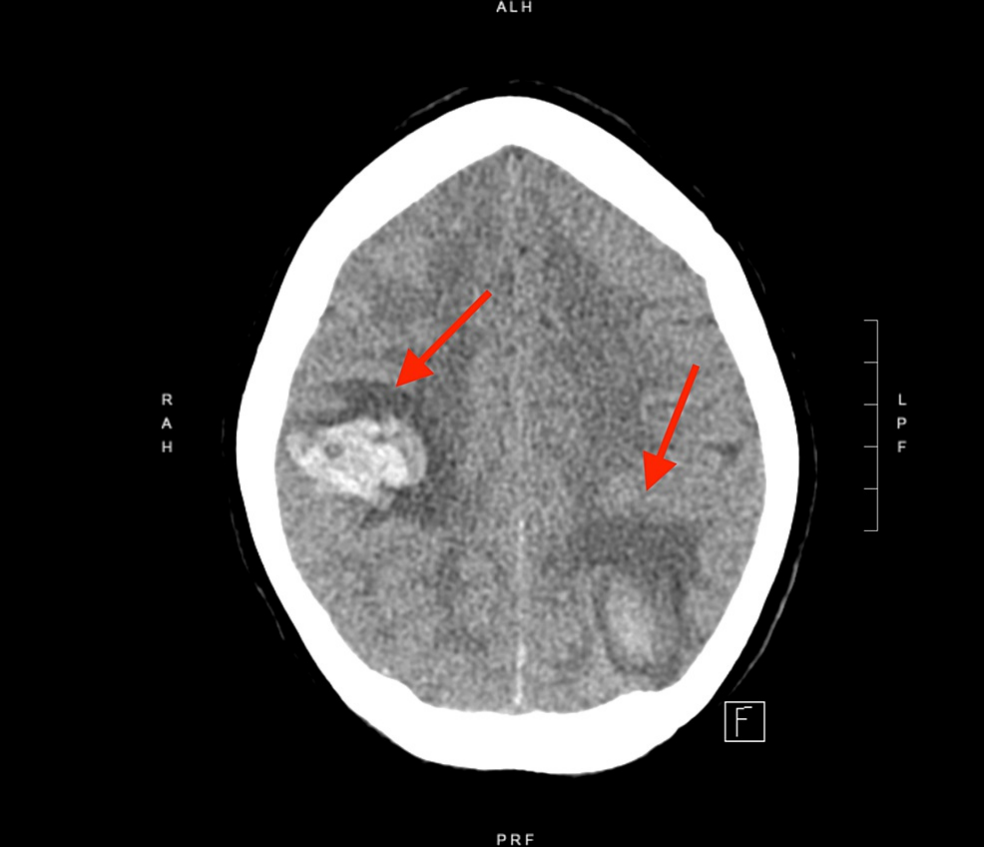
CT scan of the head Red arrows depict the right and left parietal foci of hemorrhages CT: computed tomography
